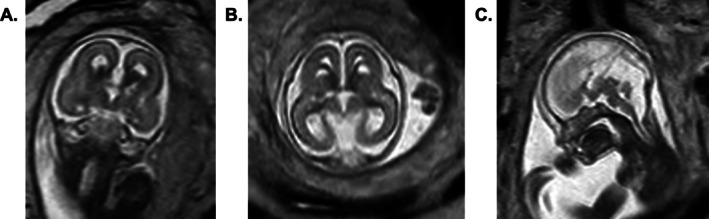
Antenatal magnetic resonance imaging (Philips GE 1.5) at 21 + 2 weeks gestation. (A) Coronal T2 demonstrating callosal agenesis, cystic spaces within the basal ganglia. (B) Axial T2 demonstrating cystic spaces within the caudothalamic eminences, thalami, and periventricular white matter. (C) Sagittal T2 demonstrating callosal agenesis and inferior vermian hypoplasia.
